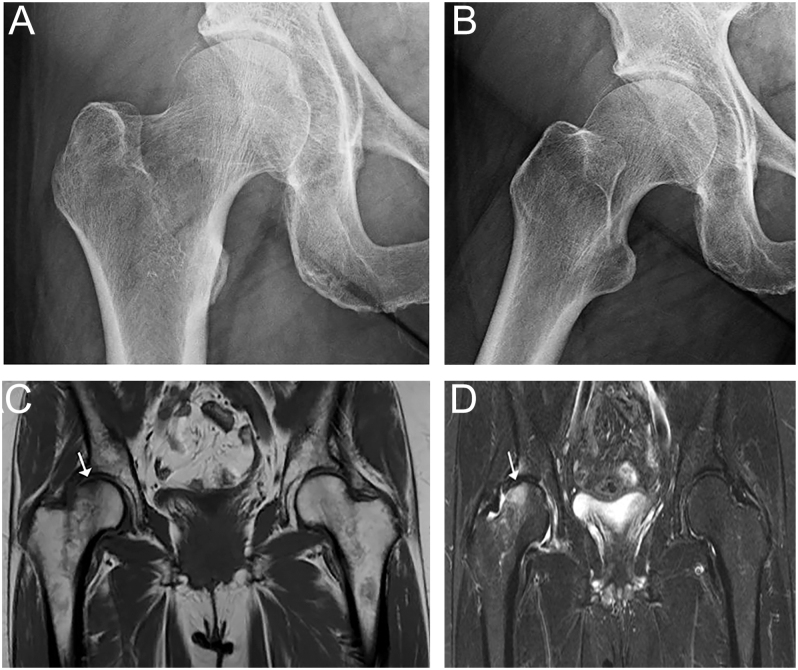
A 69-year-old female with right-sided hip pain – subchondral insufficiency fracture. AP (A) and lateral (B) radiographs of the right hip demonstrate a normal appearance of the right hip aside from regional chondrocalcinosis. Coronal T1 (C) and T2 fat-suppressed (D) MR images from MRI acquired 5 days later demonstrate marrow edema within the right femoral head with a subtle band of subchondral hypointense signal and articular surface flattening (arrows).
